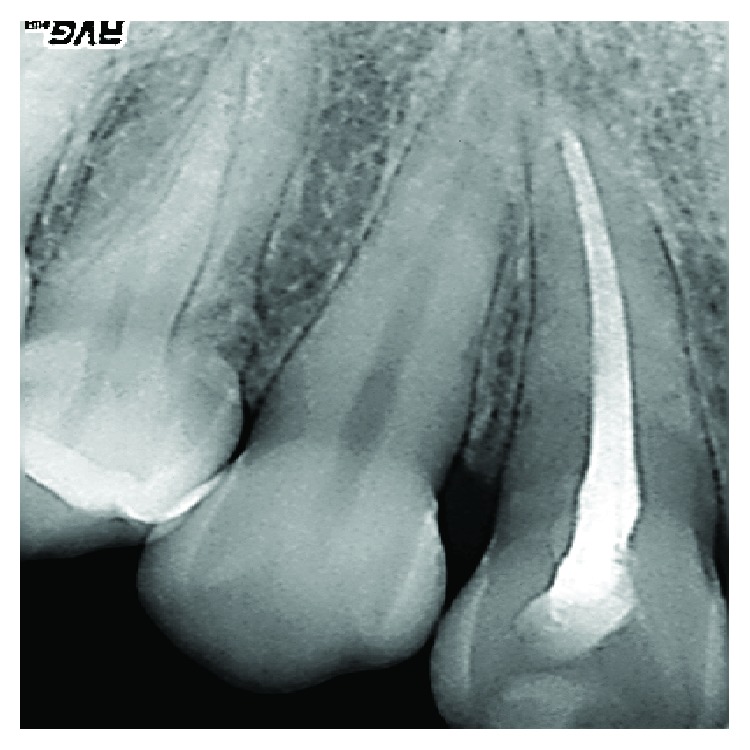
Radiographic examination after 12 months.
